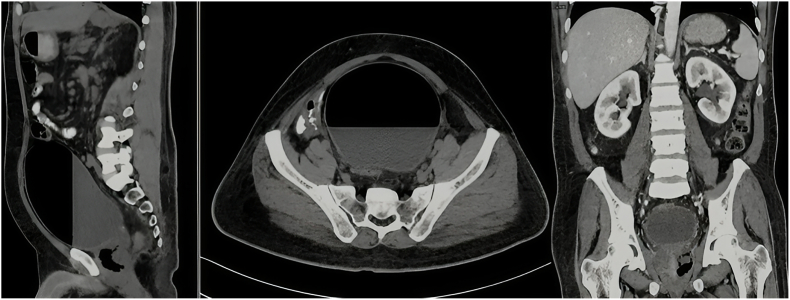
CT abdomen and pelvis with contrast revealed a multiloculated prostatic abscess measuring about 5 x 6 × 5.6 cm with intraprostatic gas collections and an air-fluid level in the urinary bladder.
